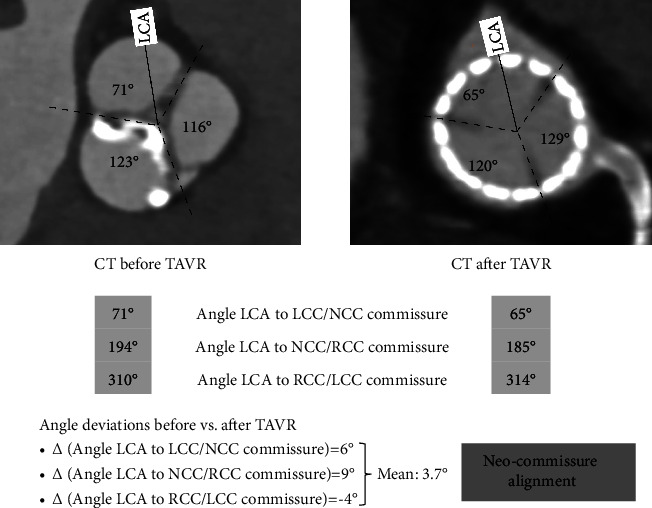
The procedure of obtaining the commissural angel deviation from CT before vs. after TAVR from case 5. LCA = left coronary artery; CT = computed tomography; TAVR = transcatheter aortic valve replacement; LCC = left coronary cusp; NCC = noncoronary cusp; RCC = right coronary cusp.
